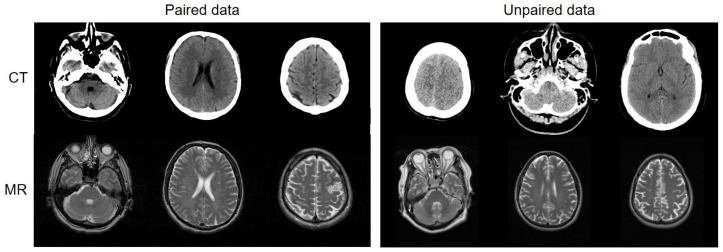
(Left): Deep networks train with paired data, which include CT and MR slices taken from the same patient at the same anatomical location. Paired data must be intentionally collected and aligned, which imposes difficulty. However, paired data provide network regression constraints that are far more correct. (Right): Deep networks train with unpaired data, which include CT and MR slices taken from different patients at different anatomical locations. There is a considerable amount of unpaired data available.
